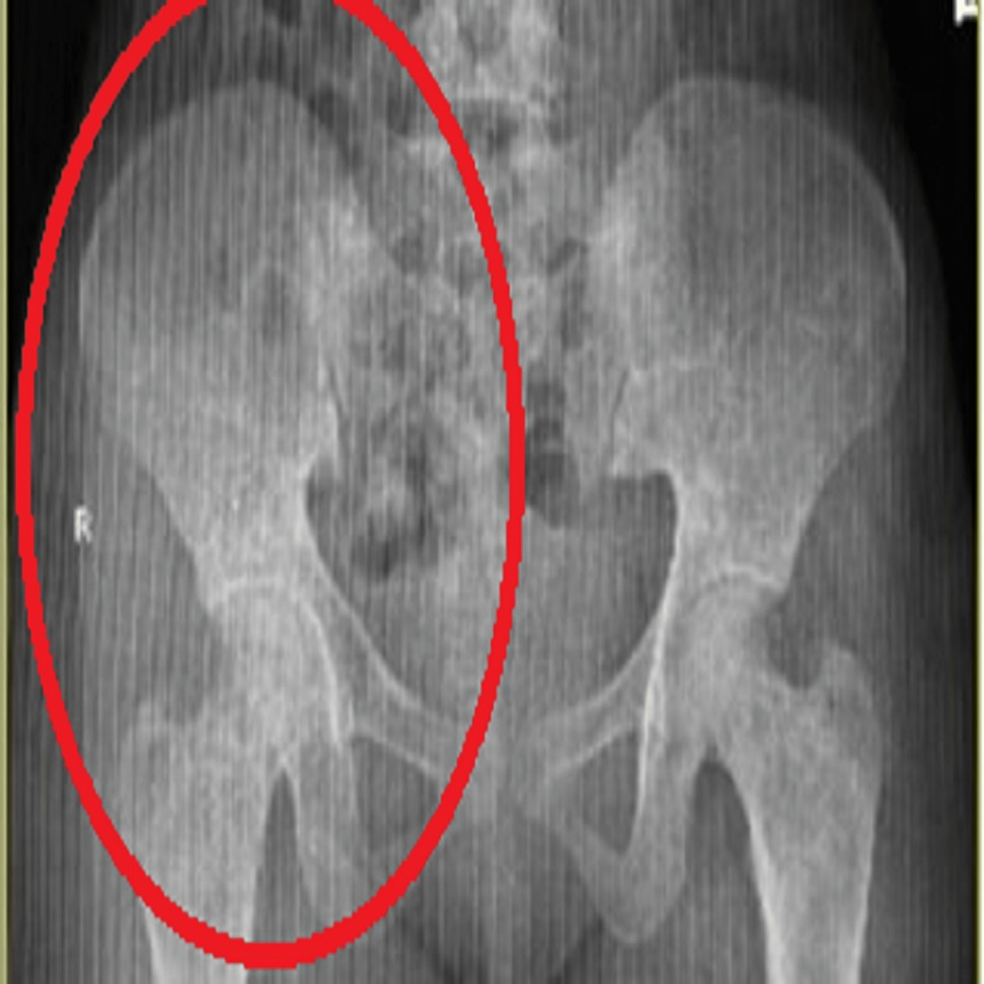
X-ray of pelvis showing lytic sclerosis
